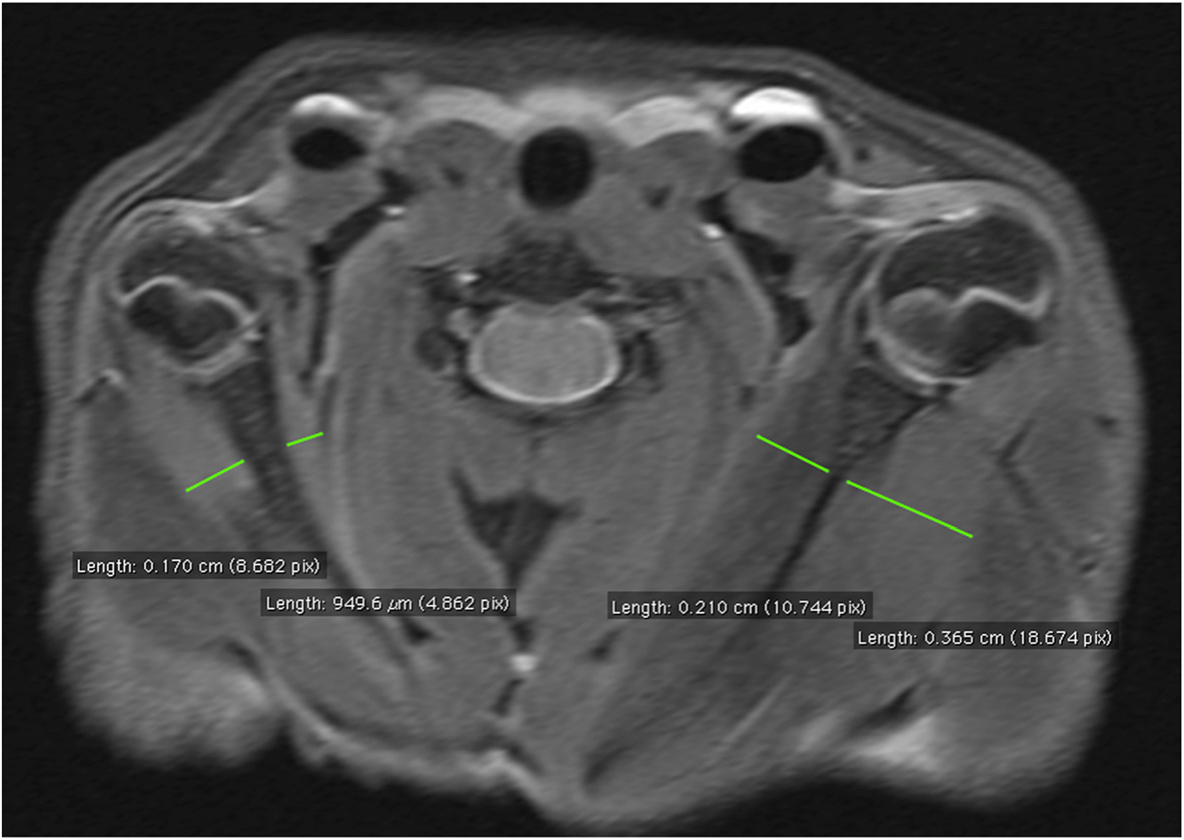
Shoulder MRI 8 weeks after surgery showing a marked atrophy of both infraspinatus and subscapular muscle that reproduces muscle changes found in children.
